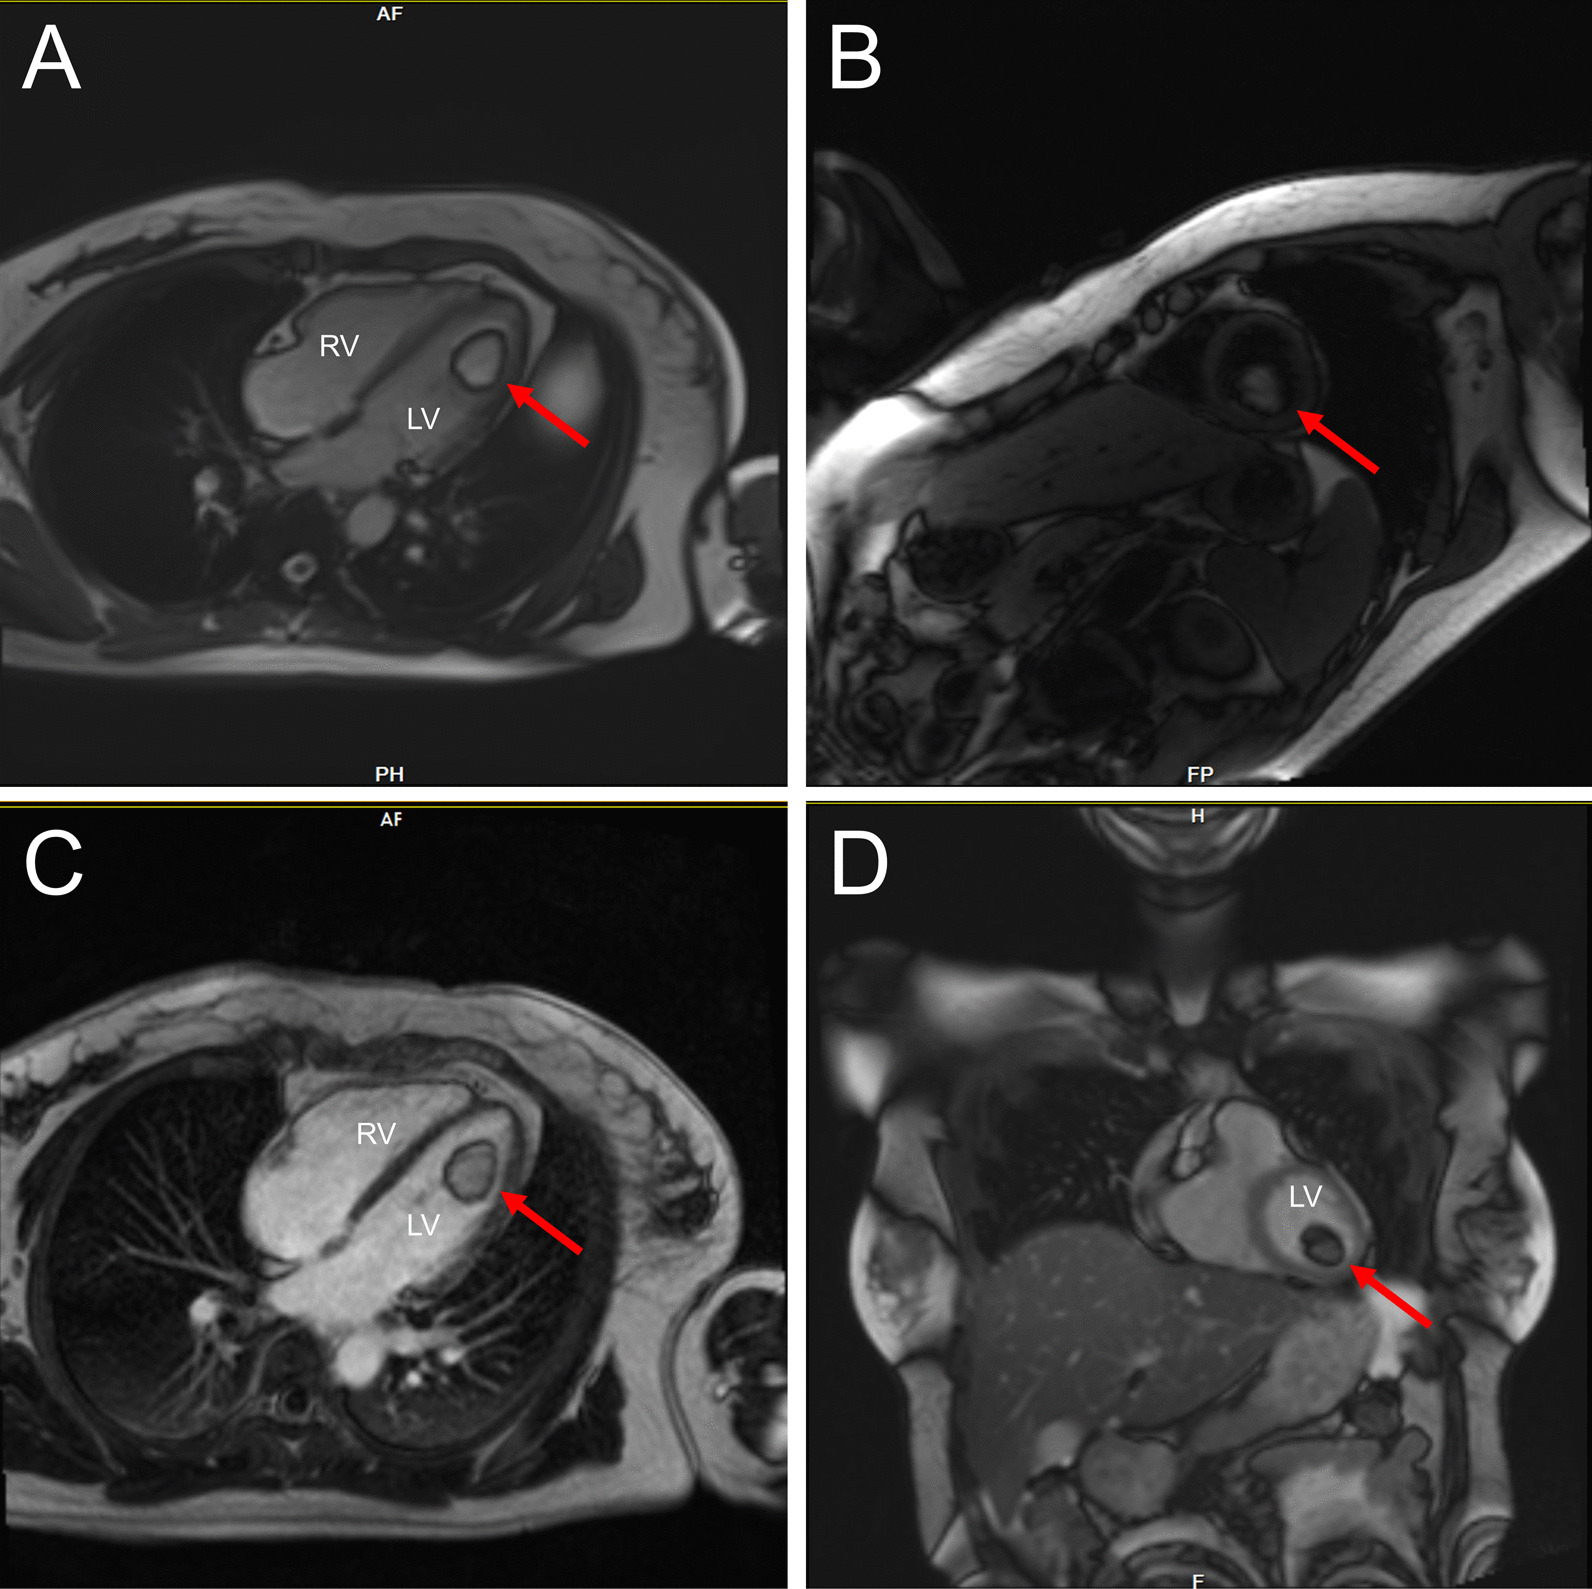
Magnetic resonance imaging confirmed a huge low signal non-enhancing lesions in the LV with a clear boundary and no obvious enhancement after perfusion (A–D). LV: left ventricle; RV: right ventricle
